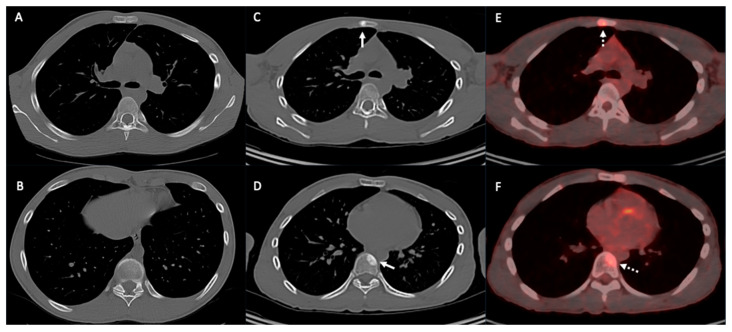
Patient with osteosarcoma and bone metastasis. Axial non-enhanced images (A,B) show no osseous lesions. Follow-up axial non-enhanced CT (C,D) and axial fused PET CT (E,F) images show an interval development of sternal and vertebral sclerotic lesions (arrows) with FDG avid uptake (dashed arrows) in keeping with metastasis.
